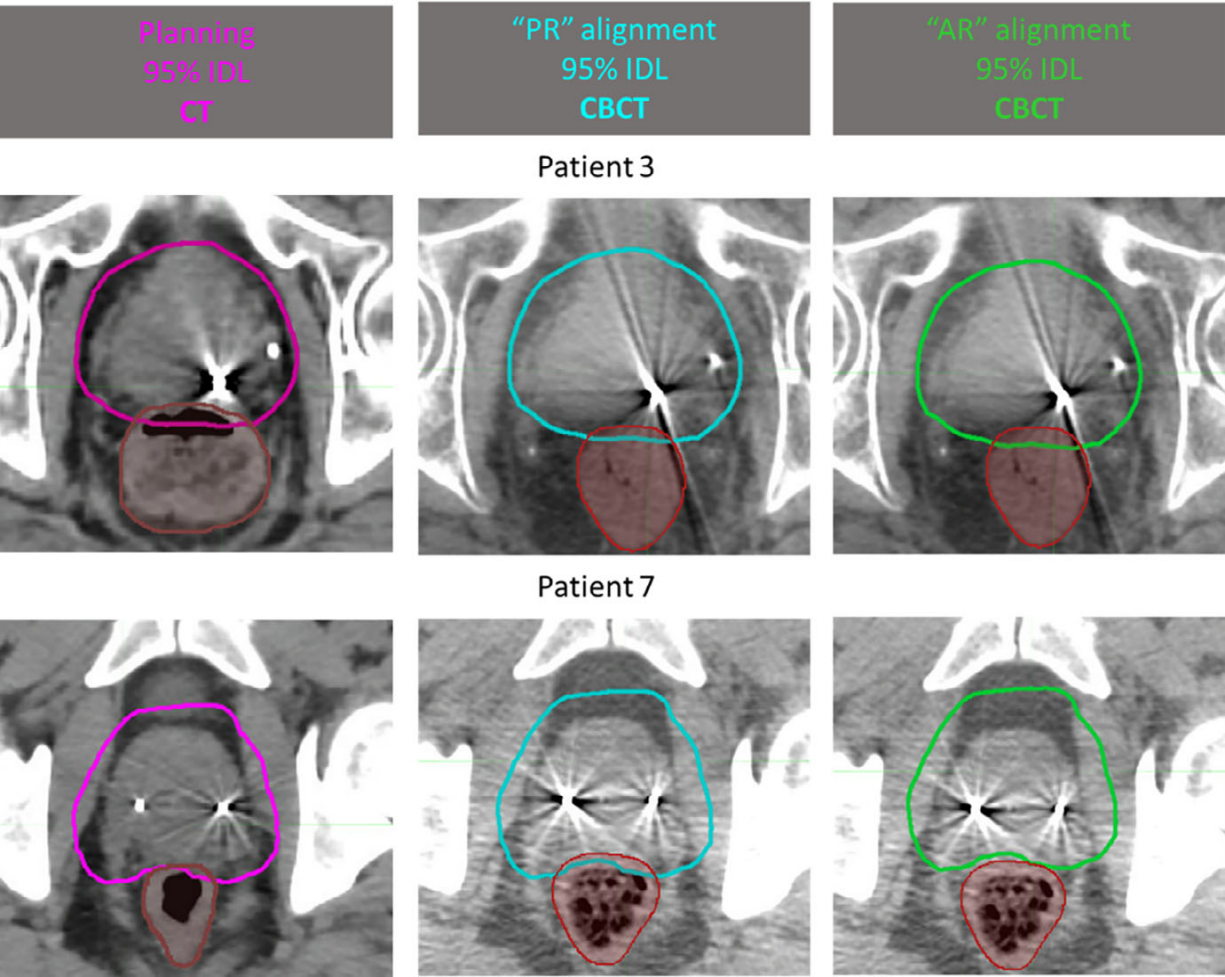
Example “PR” versus “AR” alignments with 95% IDL projected on the planning CT and daily CBCT. For the “PR” alignment (teal), the marker seeds are always at about the same location relative to the 95% IDL as the planning anatomy (pink). Brown—Rectum contour on the planning CT. Red—Rectum contour on the CBCT. Patient 3: IDL95% = 62.7 Gy. The rectum of the day as seen on CBCT is less distended compared to planning, thus further away from the prostate—the anatomy of the day is *favorable*, compared to planning. “PR” alignment: rectum receives less dose compared to planning. “AR” alignment: the anatomy has to be shifted anteriorly from the “PR” alignment and the *anterior* border of the prostate is *underdosed* compared to planning. Rectum receives more dose if “AR” alignment is executed, compared to “PR”. Patient 7: IDL95% = 57 Gy. The rectum of the day as seen on CBCT is more distended compared to planning, thus closer to the prostate—the anatomy of the day is *unfavorable*, compared to planning.“PR” alignment: rectum receives more dose compared to planning. “AR” alignment: the anatomy has to be shifted posteriorly from the “PR” alignment and the *posterior* border of the prostate is *underdosed* compared to planning. Rectum receives less dose if “AR” alignment is executed, compared to “PR”. IDL, isodose lines.
